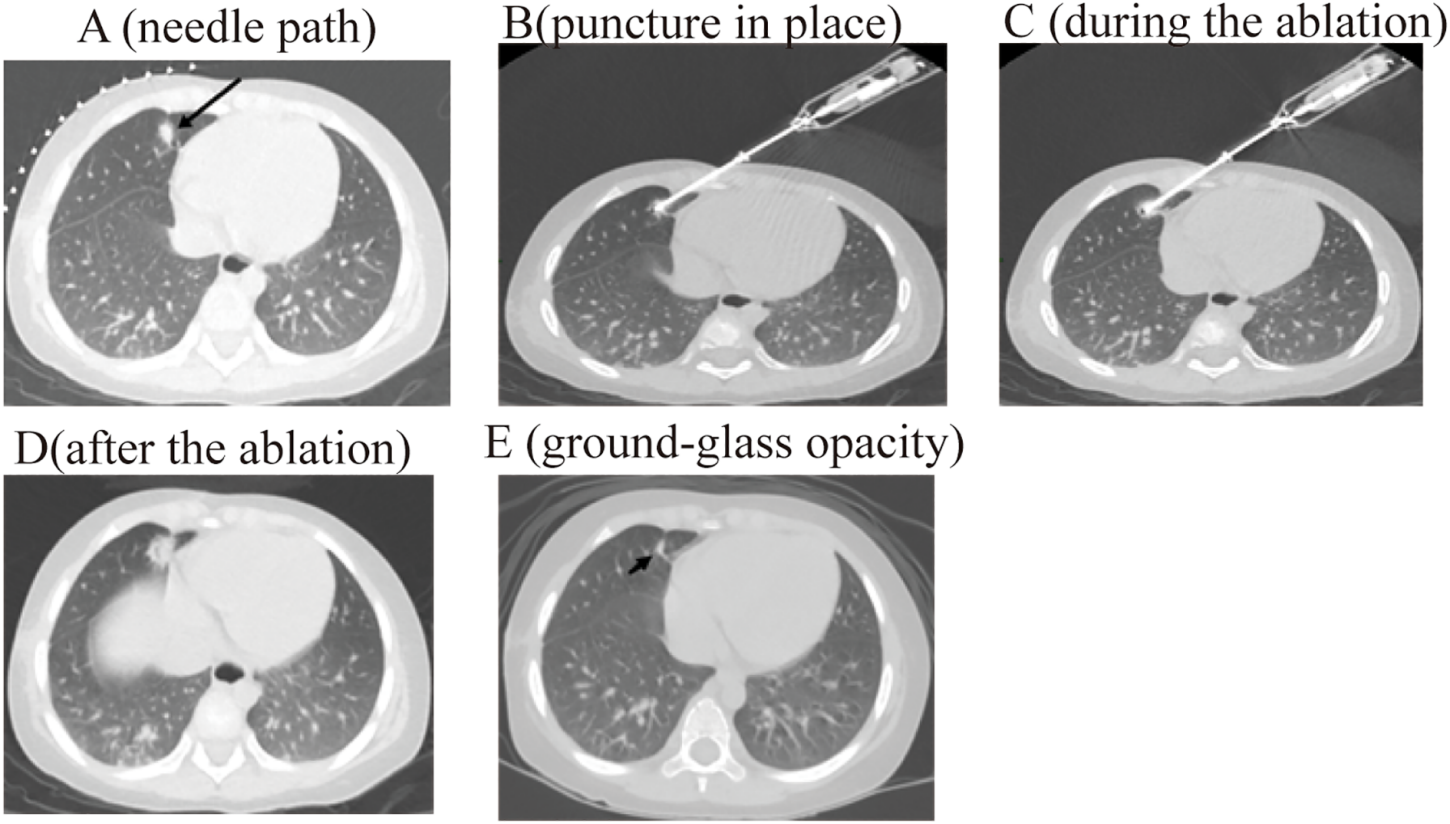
The process of radiofrequency ablation of pulmonary metastases of pediatric hepatoblastoma under CT guidance. .
